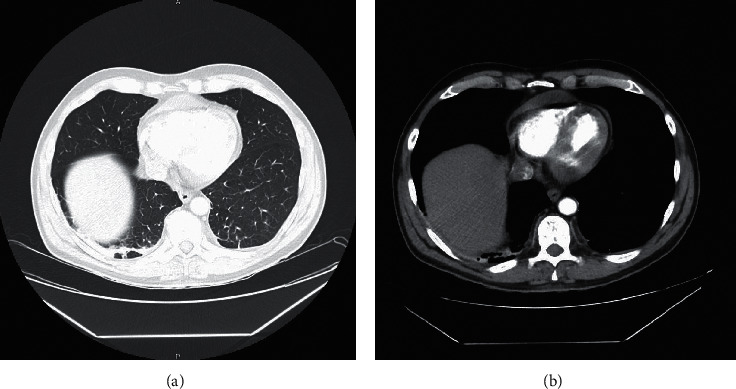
CTA chest with (a) and without (b) contrast enhancement demonstrating the moderate pericardial effusion. No pleural effusion or other abnormalities are present.
